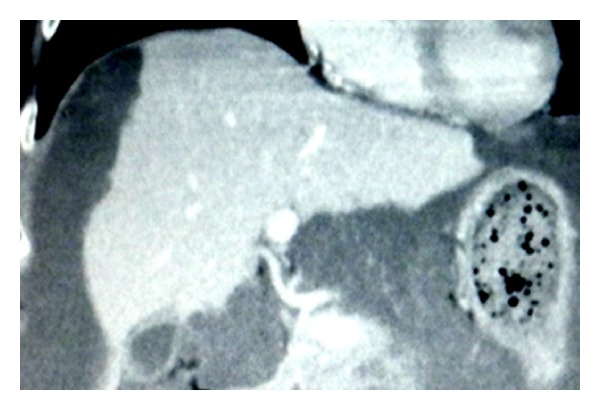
Enhanced CT scan shows tumor located in the hilar, cystic, and umbilical plate, and tumor extended in Glisson's capsule.
